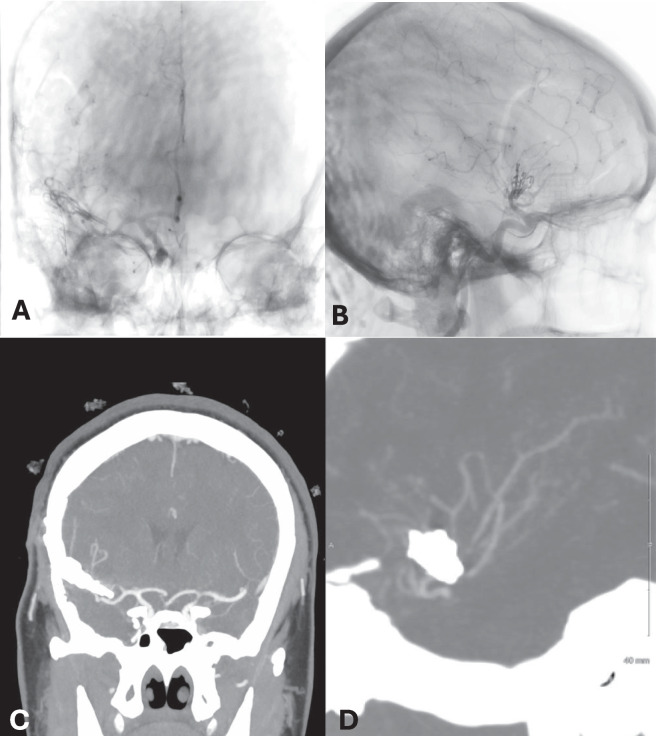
Post-operative DSA demonstrates complete preservation of flow of the reconstructed superior M2 division without luminal compromise or irregularity (A-B). 2-year post-operative coronal (C) and sagittal (D) CTA demonstrated preservation of the parent and daughter vasculature with no evidence of aneurysm recurrence
